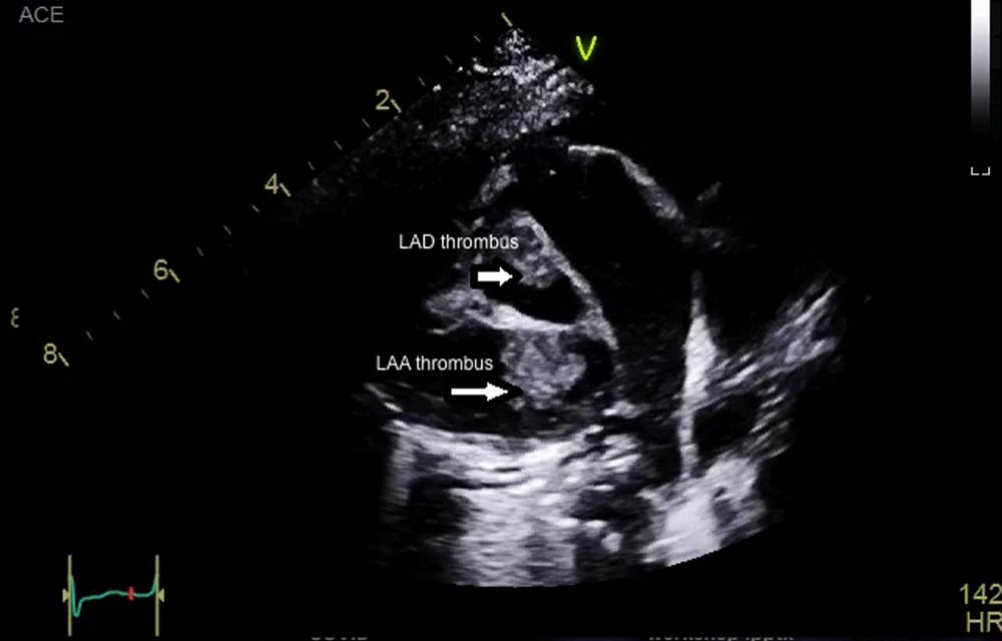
Echocardiography, long-axis view with anterior tilt, demonstrating thrombus at the left anterior descending and left atrial appendage. Arrows highlight the mural thrombus within the left anterior descending artery and the left atrial appendage.
